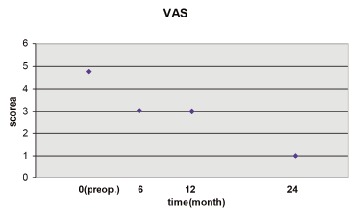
Fig. 5: Intraoperative complications, an intra-articular fracture of the lateral tibial plateau was observed on a postoperative radiograph (Picture A), which delayed weight bearing. Ten week later, fracture had complete bony union and widening medial compartment on radiograph (Picture B). Postoperative radiography of a left knee 12 months postoperatively. Bony consolidation of the osteotomy gap with remodeling is visible (Picture C). F5:
